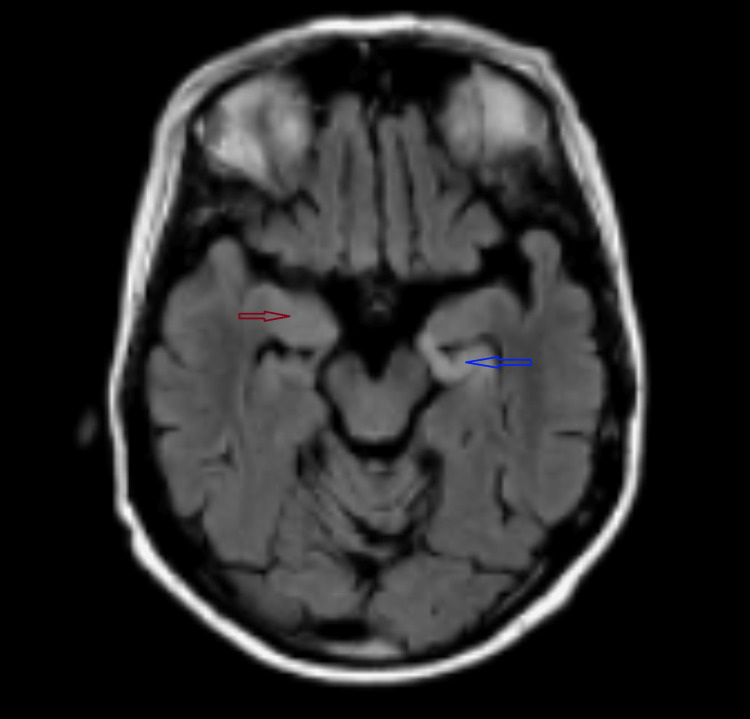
MRI brain - FLAIR sequence MRI of the brain shows T2/fluid attenuated inversion recovery (FLAIR) hyperintensity throughout the left hippocampus (blue arrow) and right amygdala (red arrow) with atrophy of the right hippocampus. There are no contrast-enhancing lesions.
